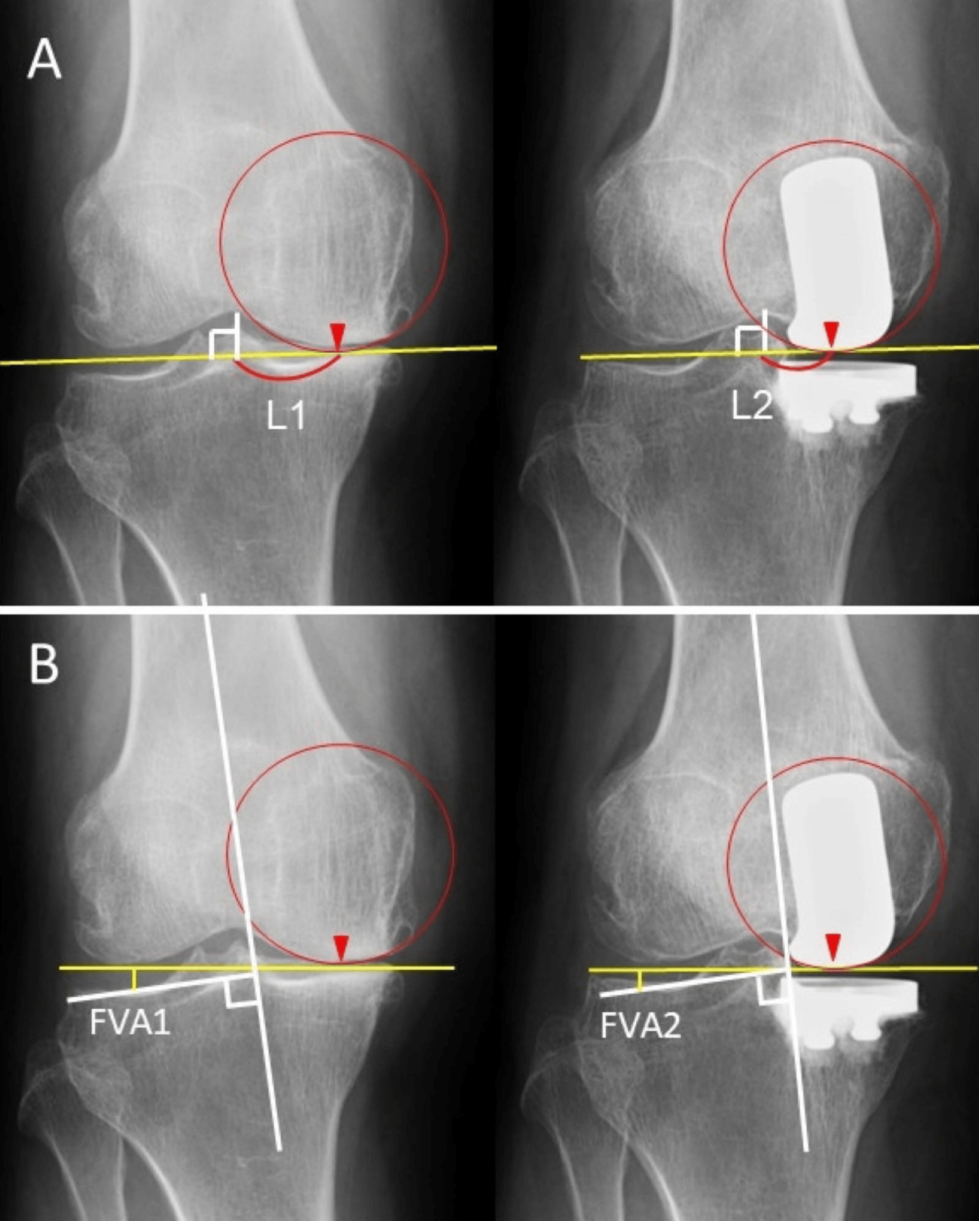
The parameters to evaluate femoral component positions The radiographic images show two parameters to evaluate femoral component positions. The apex of the medial femoral condyle was defined as the lowest point of a circle fitted to the condyle (▼). (A) Lateral shift of the medial condyle is defined as the distance between the apex of the condyle and a line passing through the apex of the intercondylar fossa and perpendicular to the joint line. (B) Femoral valgus angle (FVA) is defined as the angle between the line connecting the medial and lateral femoral condylar apexes and the femoral shaft axis. FVA1 and L1 represent preoperative values, and FVA2 and L2 represent postoperative values.
